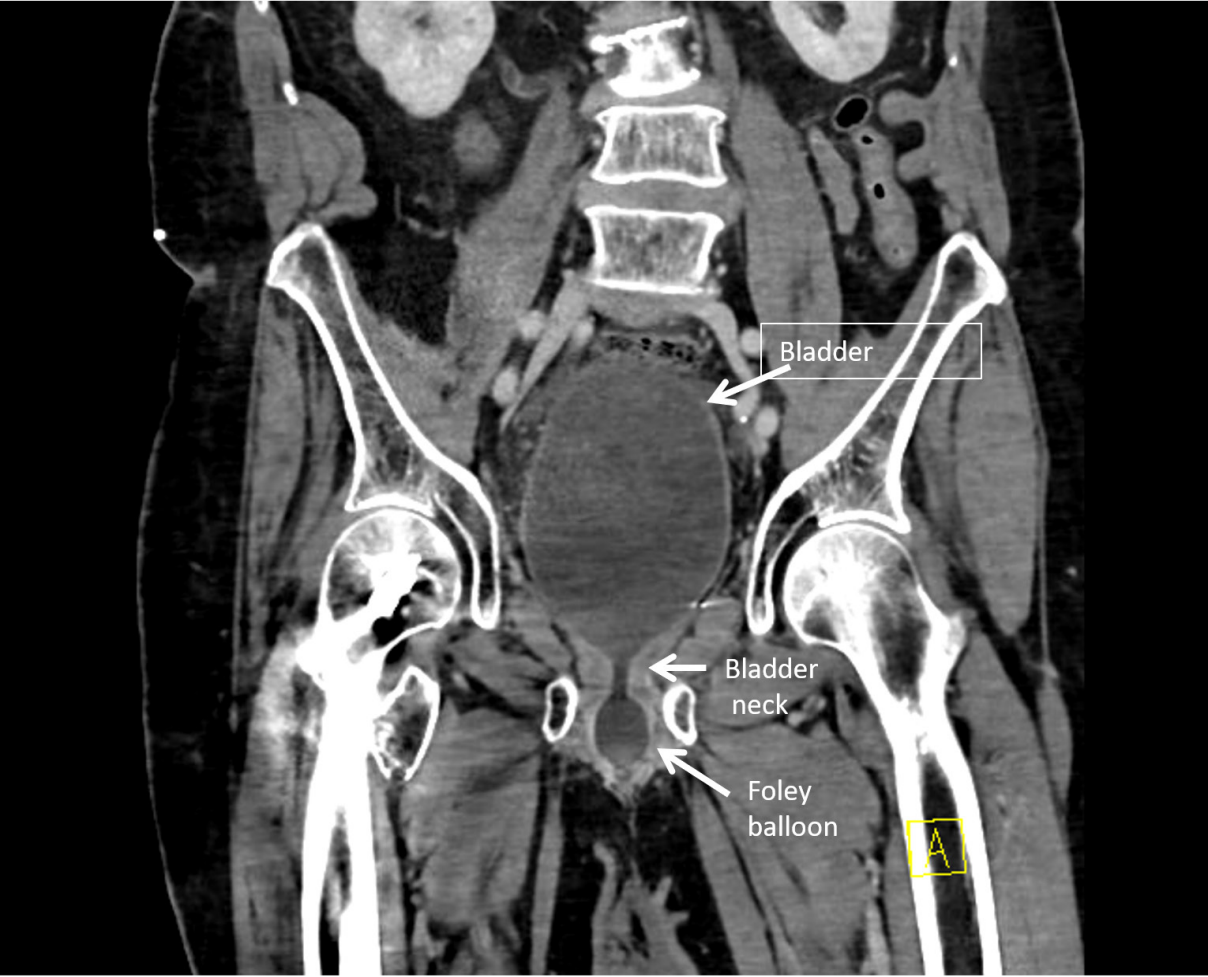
A coronal computed tomography abdomen and pelvis with intravenous contrast demonstrates a suprapubic catheter with inflated Foley balloon at the bulbar urethra caudal to the urethral anastomosis. There is also bladder distension and mild hydronephrosis from functional urinary obstruction due to malpositioned Foley balloon.
